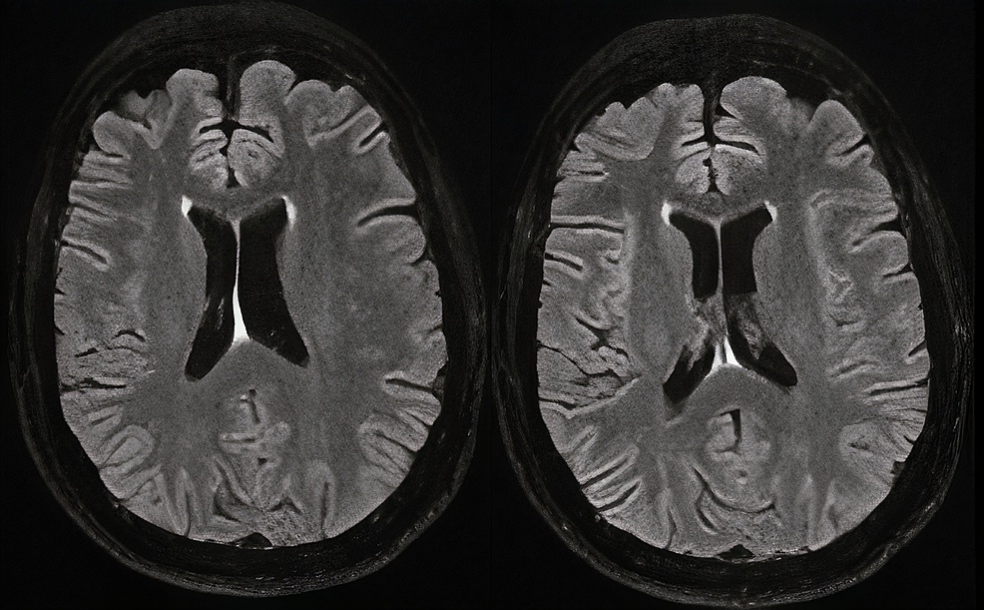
Fluid-attenuated inversion recovery (FLAIR) magnetic resonance imaging performed one month later Total regression of the FLAIR signal abnormalities
